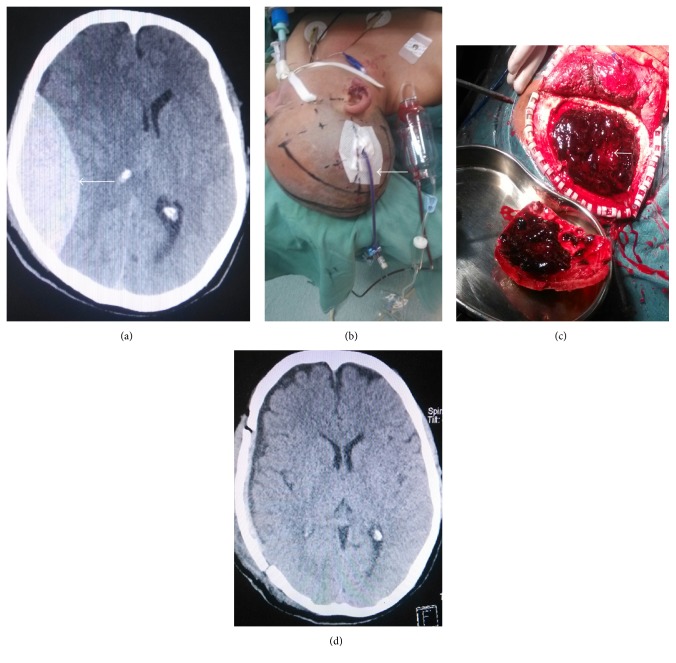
(a)–(d) Head CT images showing a huge acute epidural hematoma with cerebral hernia at the right temporal-parietal region prior to and after surgery. (a) Head CT scan image prior to surgery; hematoma volume was approximately 145 mL and midline shift 13 mm. (b) Hematoma volume with rapid minimally invasive aspiration under local anesthesia was approximately 40 mL; drainage tube was placed. (c) Skull bone flap was opened during surgery; an arrow indicates a partly aspirated hematoma in the hematoma center. (d) Head CT scan reexamined the day after surgery; drainage of hematoma was almost complete, brain tissues were expanded again, midline shift was improved, dilated pupil is recovered and light reflection was recovered.
